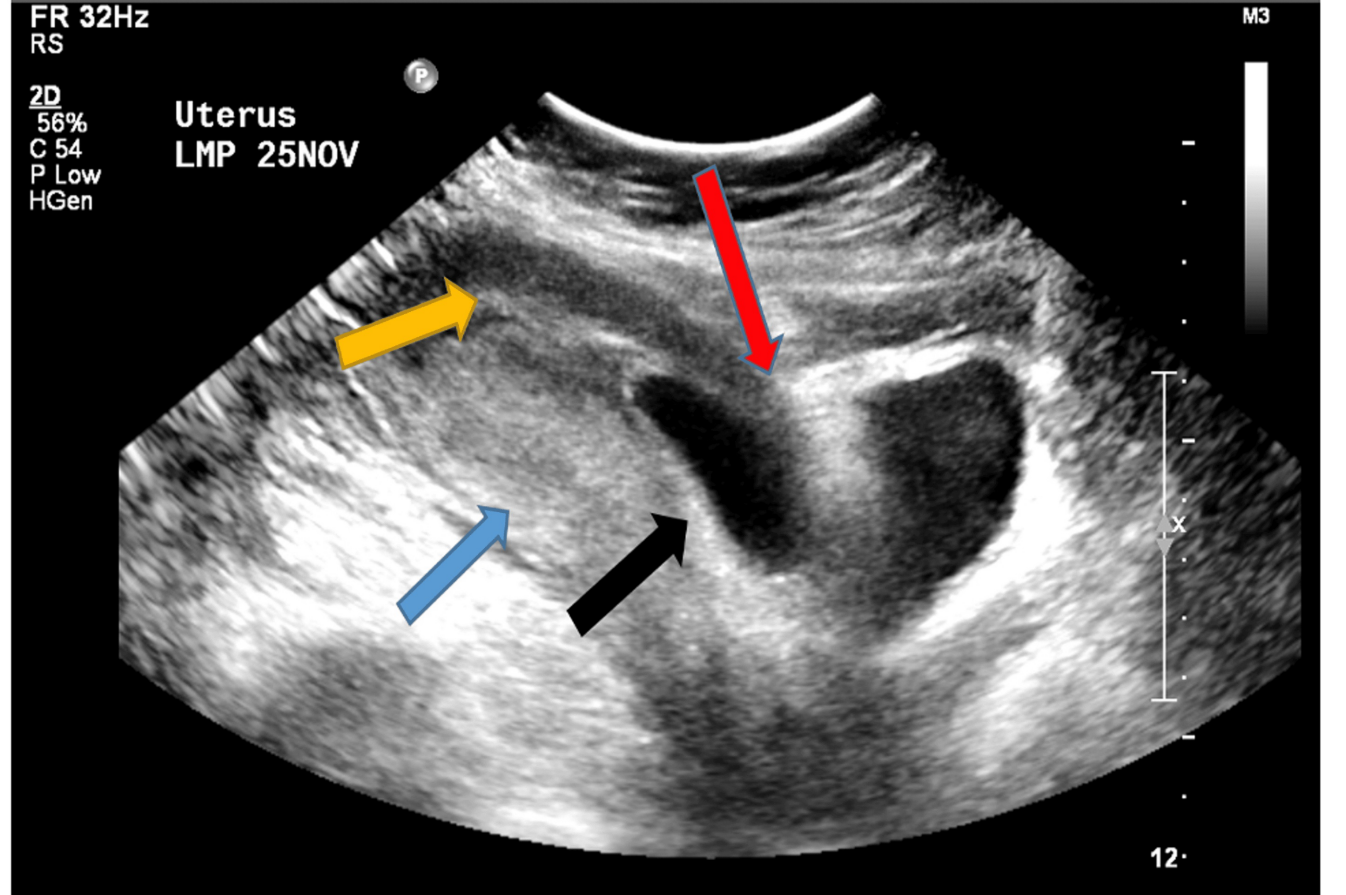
Sagittal transabdominal ultrasound showing an ectopic cesarean scar pregnancy The black arrow indicates a low-lying empty gestational sac with implantation in the area of the cesearean (CS) scar and thinning of the anterior aspect of the myometrium (red arrow). The yellow arrow indicates an empty endometrium. The blue arrow indicates the normal thickness of the posterior myometrial wall.
